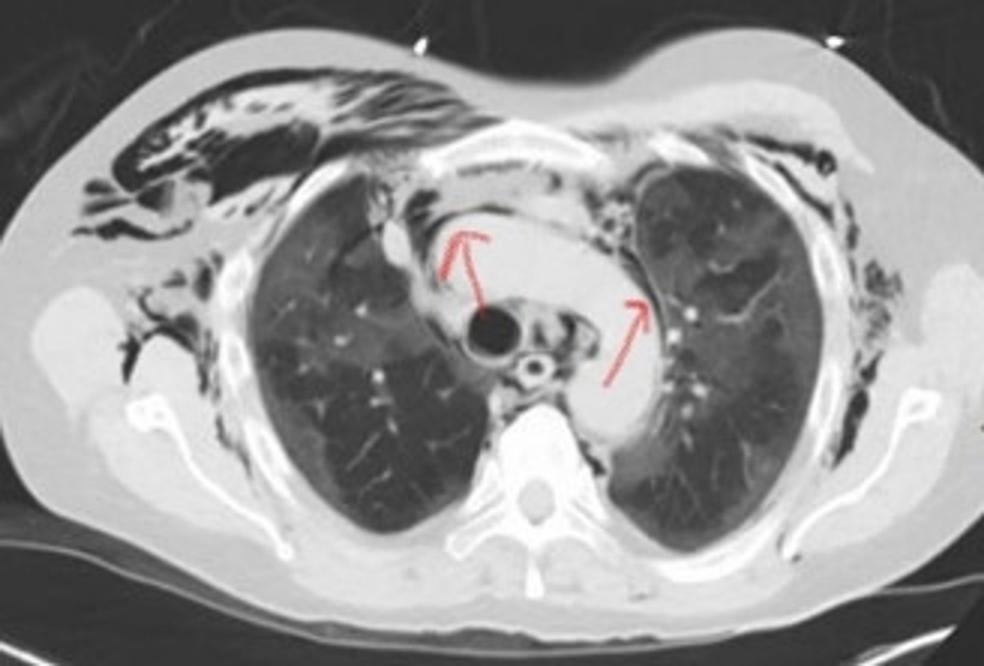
CT chest showing large pneumomediastinum, pneumopericardium, and extensive chest wall and bilateral neck base subcutaneous emphysema
